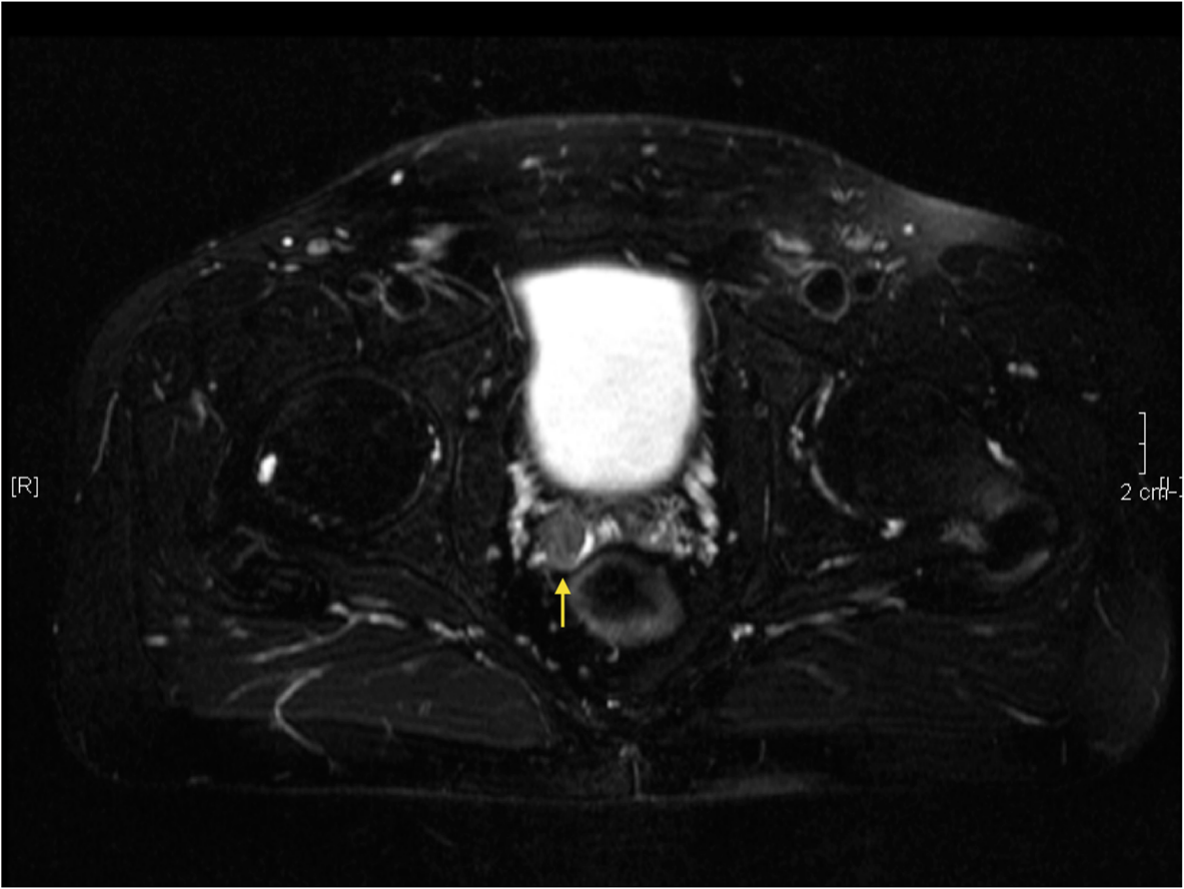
Axial T2-weighted MRI of the right seminal vesicle mass.
